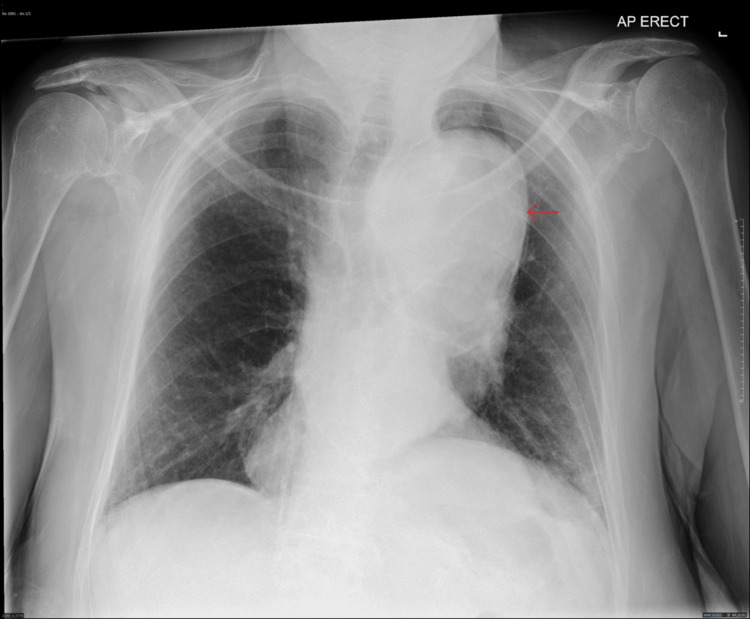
Chest X-ray A large thoracic aortic aneurysm was found in the region of the aortic arch, which was not evident on previous examination as highlighted by the red arrow.
